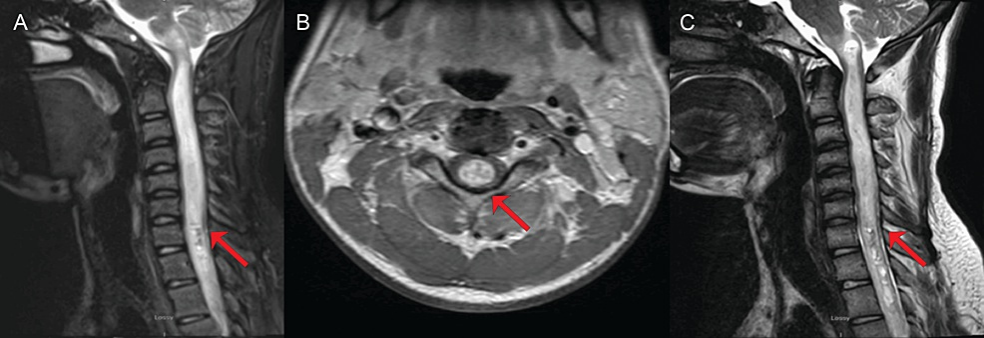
Magnetic resonance imaging of the cervical spine shows the expansile, heterogeneously enhancing intramedullary lesion (A) Sagittal T2-weighted MRI shows a large, hyperintense intramedullary lesion extending from the C1 to T3 vertebral. (B) Axial T2-weighted MRI shows an expansile, hyperintense intramedullary lesion. (C) Follow-up sagittal T2-weighted MRI shows the rostral extension of the lesion into the brainstem with increased surrounding edema. Red arrows show the lesions
